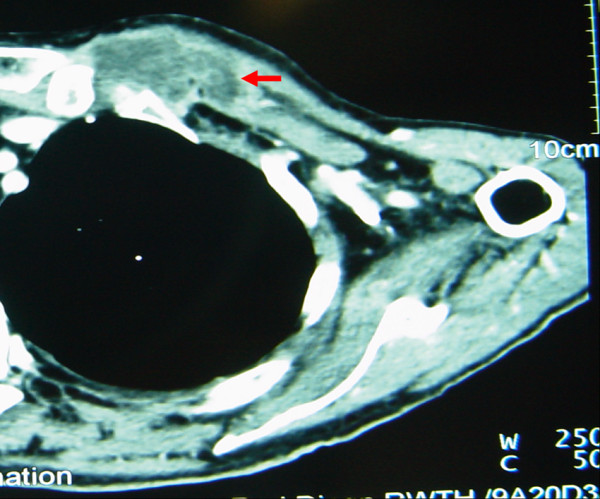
Contrast enhanced CT, soft tissue window: Soft tissue metastasis at the left upper ventral throracic wall.
